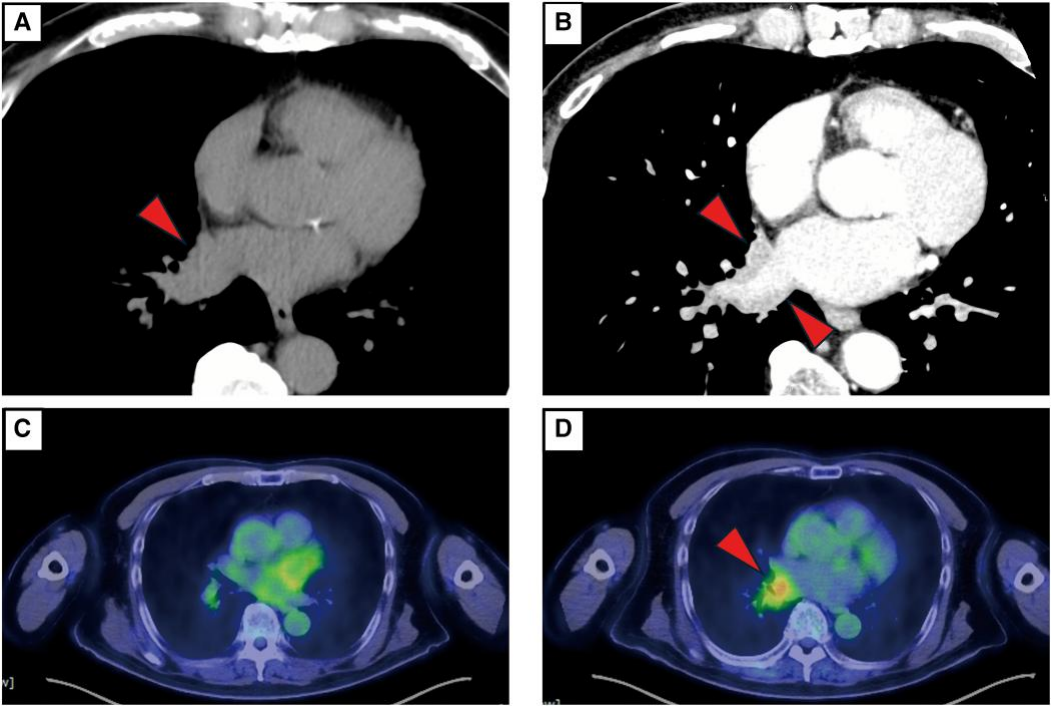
Multimodal imaging findings. (*A*) Retrospective review of follow-up non-contrast computed tomography revealing subtle thickening near the right inferior pulmonary vein. (*B*) Pre-ablation contrast-enhanced computed tomography demonstrating a soft tissue lesion extending into the right inferior pulmonary vein and adjacent left atrial wall, suggestive of lymphoma recurrence. (*C*) Positron emission tomography–computed tomography during remission showing no abnormal FDG uptake in the right inferior pulmonary vein region. (*D*) Post-ablation Positron emission tomography–computed tomography demonstrating intense FDG uptake (SUV 5.11) in the right inferior pulmonary vein region, consistent with metabolically active tumour involvement.
